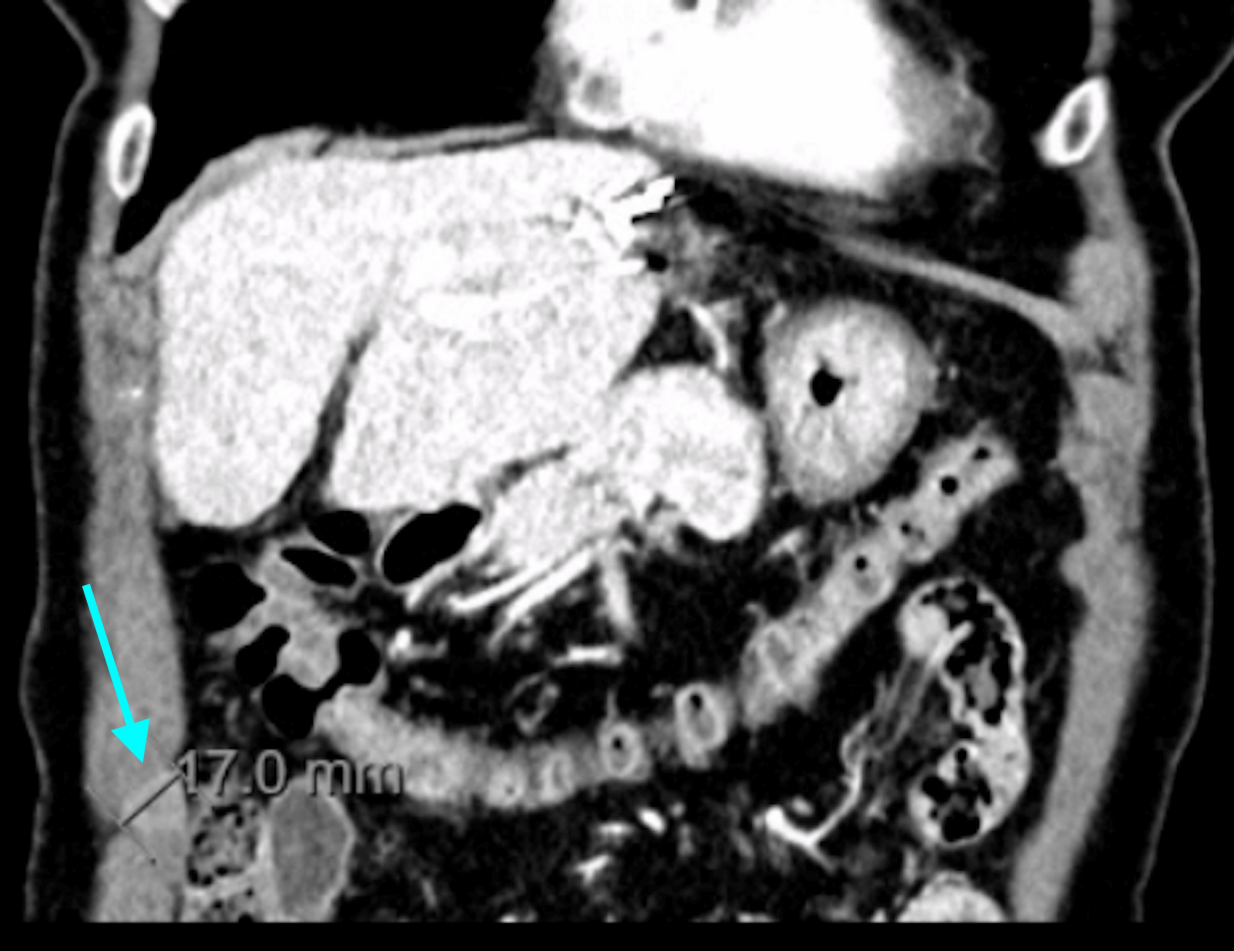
Contrast-enhanced abdominal computed tomography demonstrating a nodular lesion within the right anterolateral abdominal wall musculature, measuring approximately 17 mm, with heterogeneous enhancement and central washout in delayed phases, suggestive of malignancy
